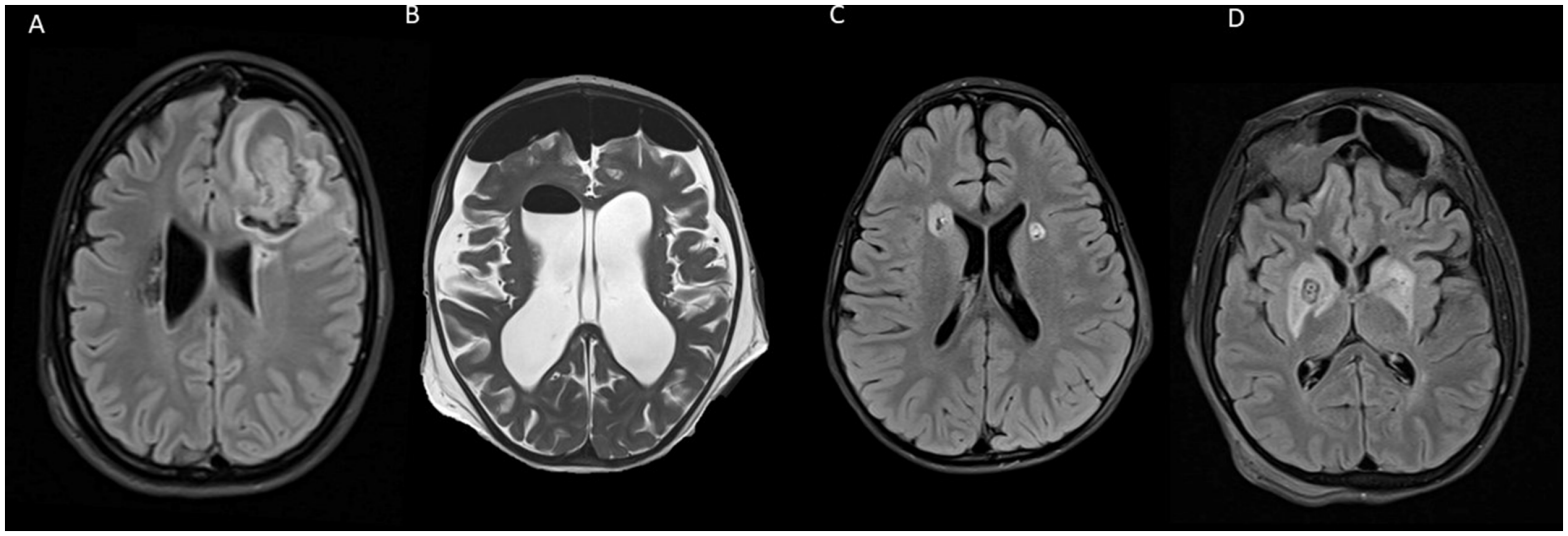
Radiologic complications after RP. (A) Frontal bleeding along the surgical trajectory. The patient presented with contralateral hemiplegia, motor apraxia, and cognitive decline. (B) Pneumoventricle and frontal pneumoencephalon. (C) White matter FLAIR hyperintensity along the surgical trajectory. (D) Diffuse oedema involving the striatal nuclei bilaterally.
